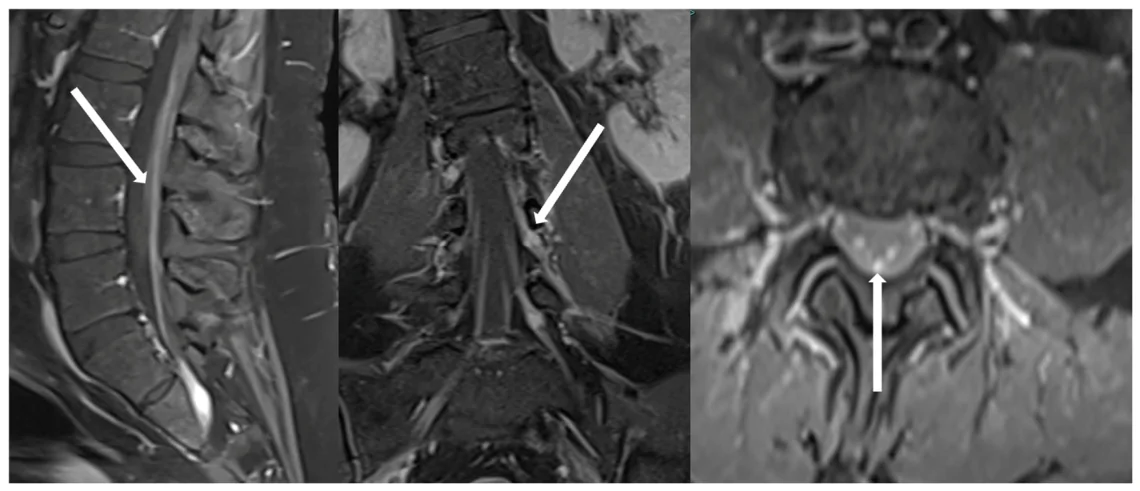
Case 3. A 52-year-old man presented with progressively worsening low-back pain and progressive symmetric lower-limb weakness and generalized areflexia. Contrast-enhanced fat-suppressed T1-weighted MRI in the sagittal, coronal, and axial planes demonstrates diffuse, smooth, and symmetric enhancement of the cauda equina nerve roots (white arrows), predominantly involving the ventral roots, contributing to the lumbosacral plexus. This pattern is suggestive of an inflammatory polyradiculoneuropathy, such as acute inflammatory demyelinating polyradiculoneuropathy, rather than an isolated lumbar plexopathy. Original Image.
